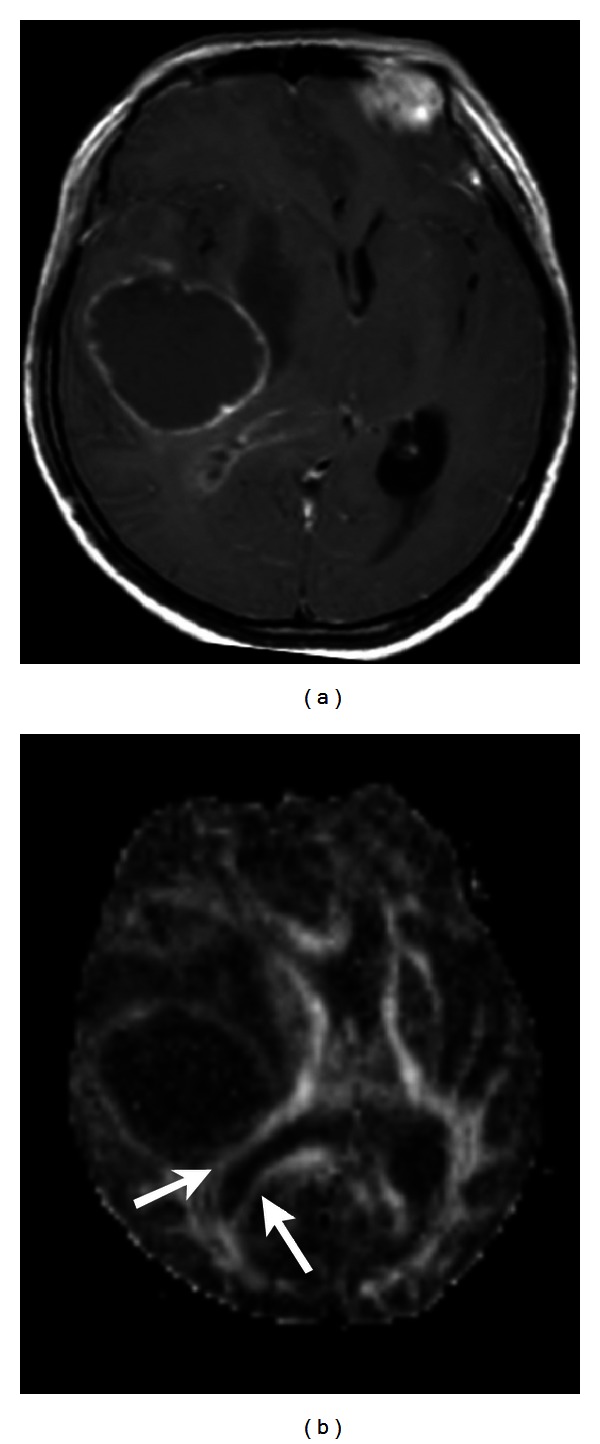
(a) Contrast-enhanced T1-weighted image shows a necrotic glioblastoma with rim-like enhancement. (b) On FA map, attenuated FA (arrows) of the adjacent tracts is shown, indicating tumor infiltration.
